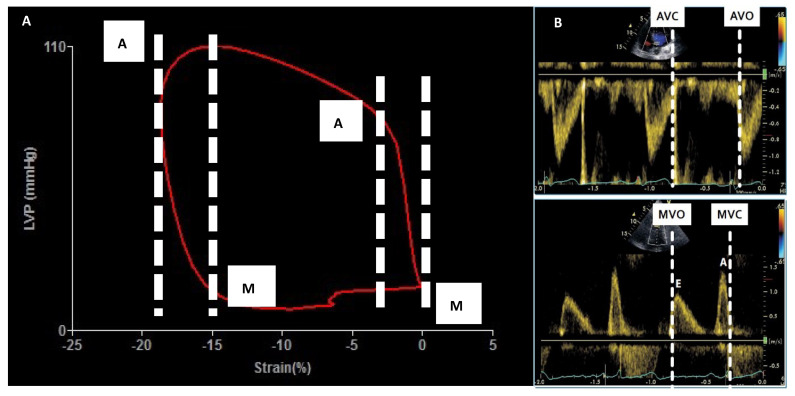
Methodology for measuring strain-derived myocardial work. In the left side the strain pressure loop: the area inside the loop corresponds to global work index (panel A). In the right panel Doppler-derived measurement needed to mark systolic and diastolic time intervals (panel B). AVC = Aortic valve closure, AVO = Aortic valve opening, LVP = Left ventricular pressure, MVC = Mitral valve closure, MVO = Mitral valve opening.
